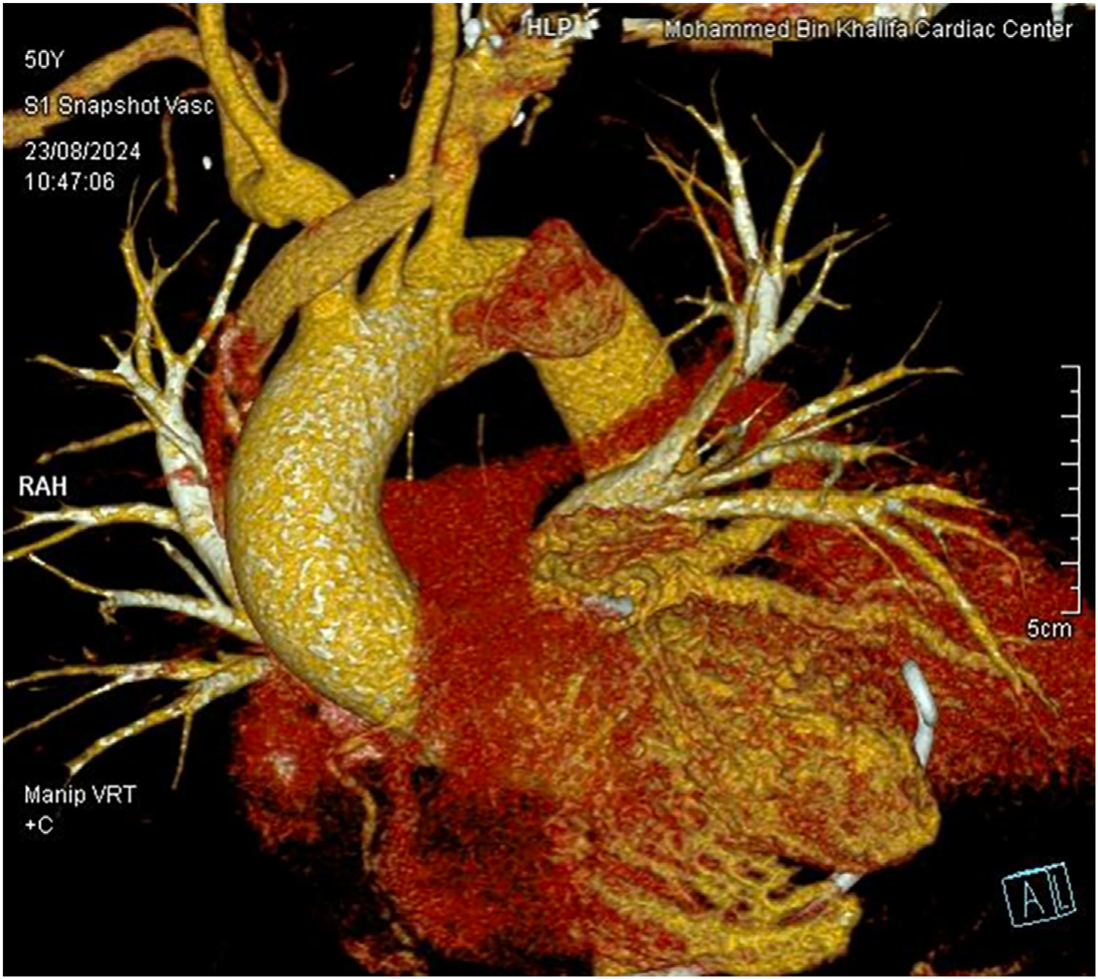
A 3-dimensional computed tomographic scan of the pseudoaneurysm. A 2.3 cm × 3 cm × 3.6 cm aortic arch pseudoaneurysm with a 13-mm was located on the anterior wall of the aortic arch at the same level as the origin of the left subclavian artery but not involving the arch vessels. (A, anterior; L, lateral; Manip VRT, manipulated volume rendering technique; RAH, right atrium of the heart; +C, contrast-enhanced.)
